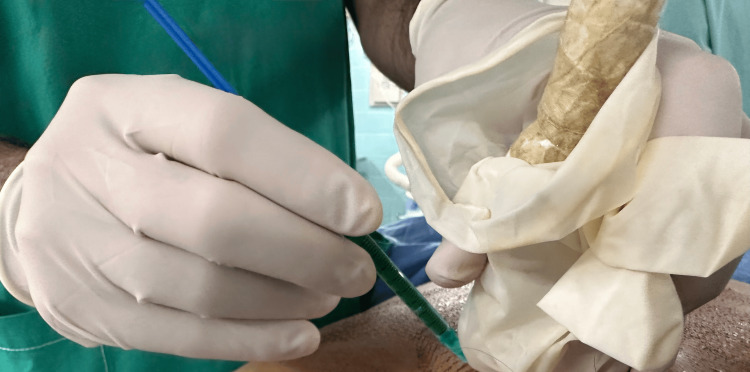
Ultrasound-guided modified dye injection 1 mL of the prepared solution was injected at the level of the target lesion.
